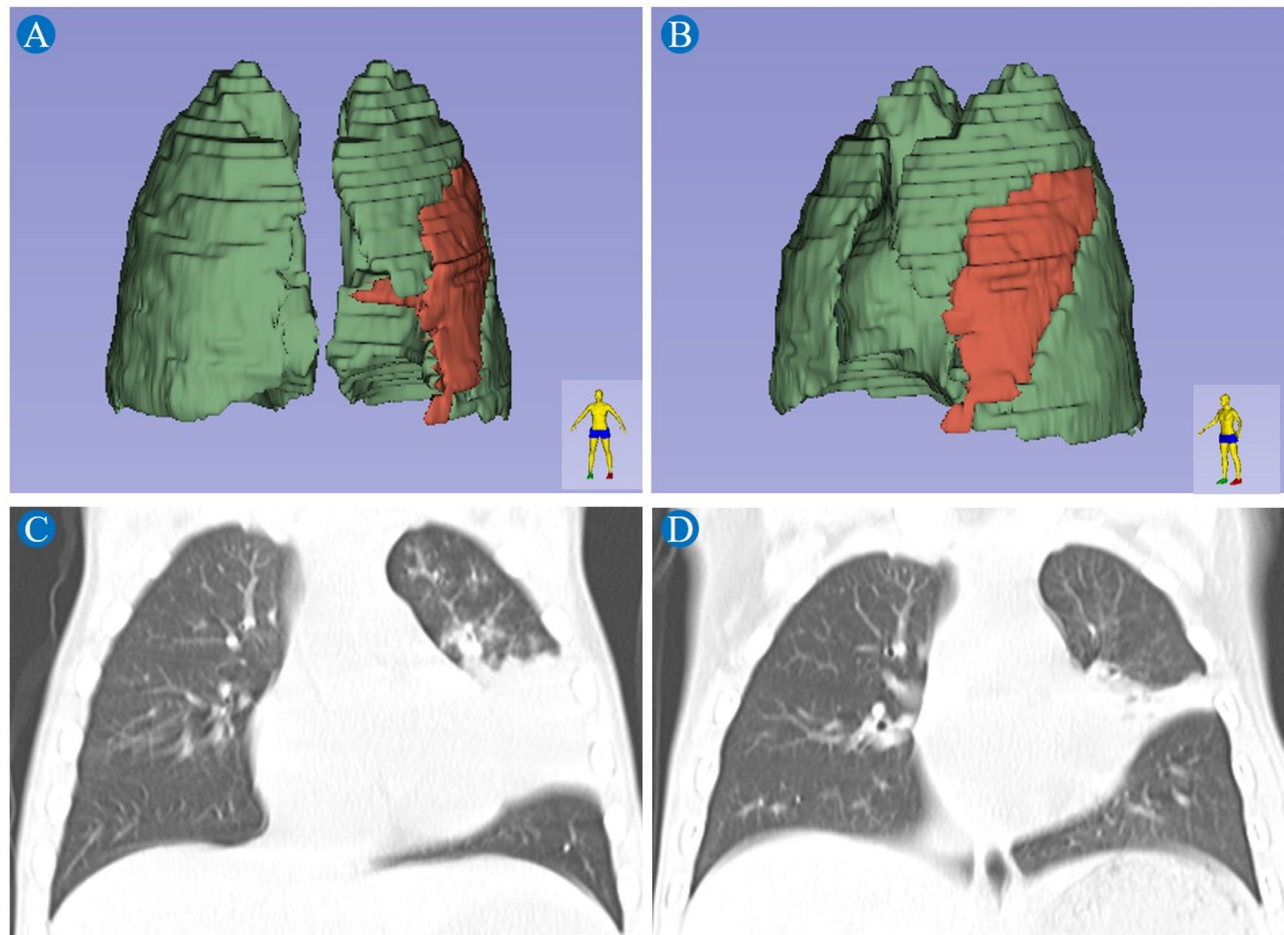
Images A-D depict a 7-year-old-boy with refractory *Mycoplasma pneumoniae *pneumonia complicated by plastic bronchitis. A Paramedian view of the lung reconstruction generated using 3D Slicer software based on manual segmentation. B Left lateral view of the affected lung, showing the distribution of diseased (red) and normal (green) tissues. The volume ratio of pulmonary lesions was calculated as the volume of diseased tissue divided by the total lung volume. C Pre-bronchoscopy chest CT (coronal view) showed extensive consolidation in the left upper lobe. D Follow-up chest CT one week post-bronchoscopy showed a patchy high-density shadow in the left upper lobe with marked radiographic improvement
